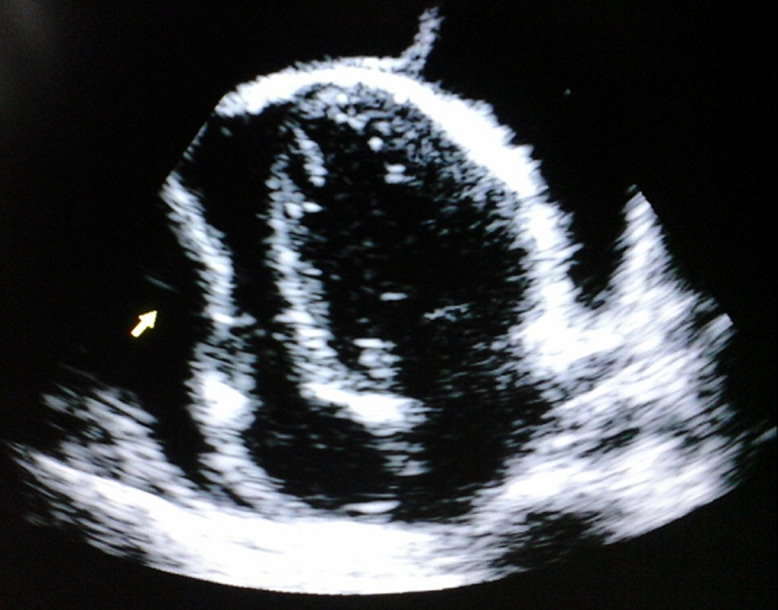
apical four chambers view showing a circumferential pericardial effusion with collapse of the right ventricle in diastole (white arrow); the surface of the heart has a shaggy appearance, with frond-like structures extending to the parietal pericardium, this appearance is typical of tuberculous pericardial effusion
